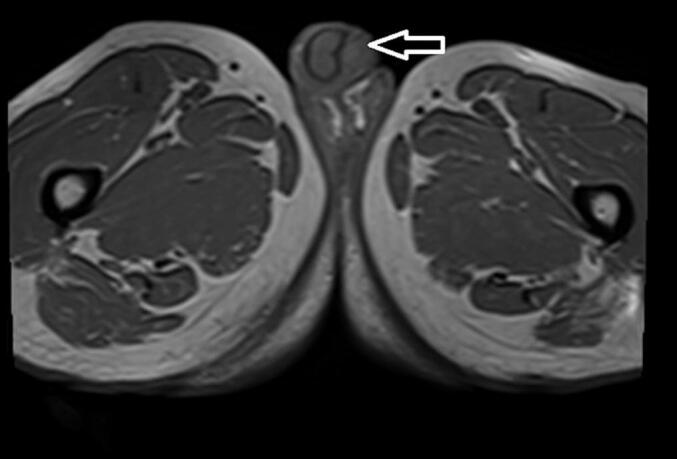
Axial pelvic MRI arrow head showing T1 intermediate intense round lesion at distal penile invading corpus spongiosum.
